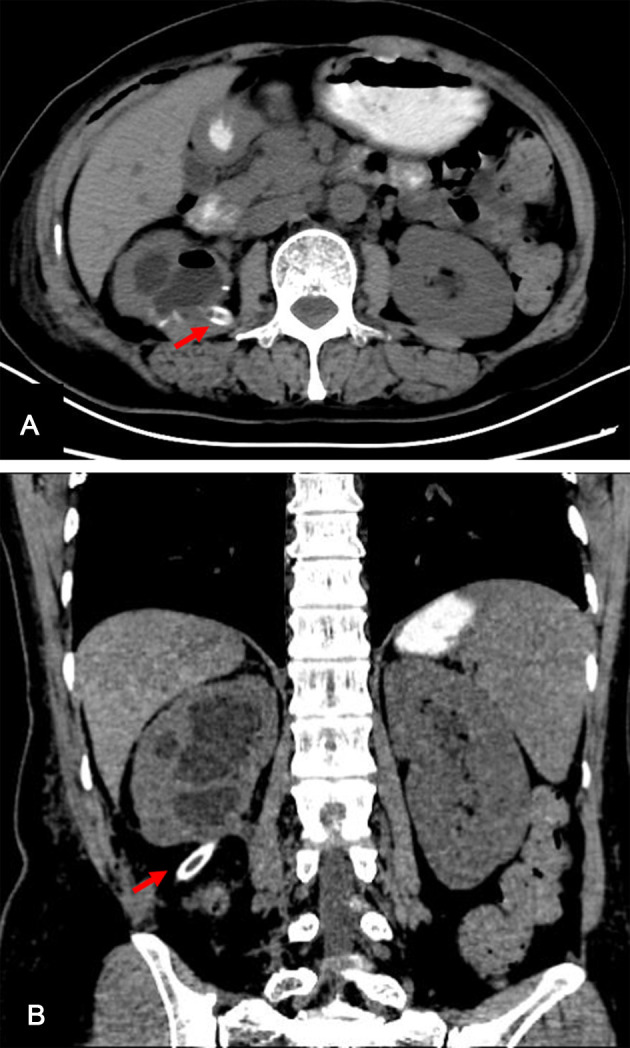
CT scan, which was performed after retaining the drainage tube for 34 days, showing no obvious perirenal exudation. The red arrow refers to drainage tube. (A) Sagittal images, (B) coronal image. CT, computed tomography.
